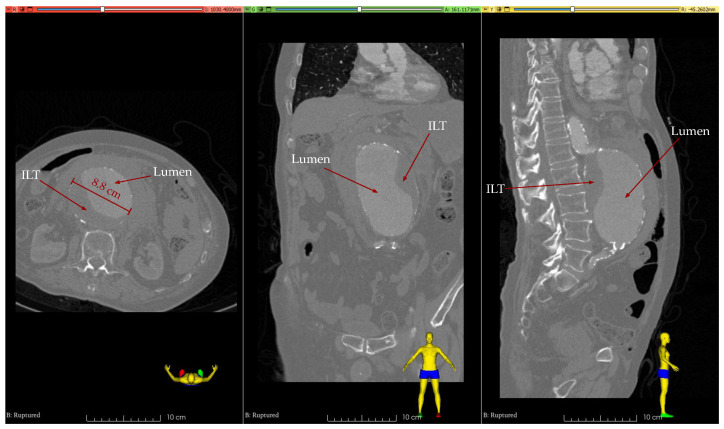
CT images after AAA rupture: axial, coronal, and sagittal views (from left to right).
